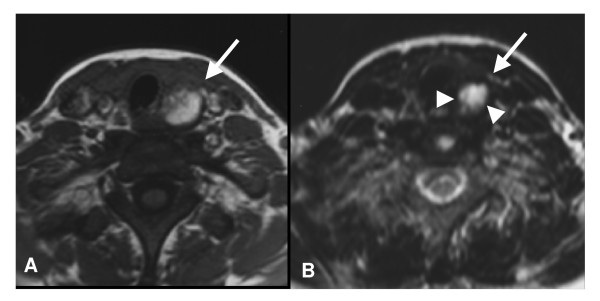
Left inferior parathyroid adenoma. Axial MR imaging scans (07/1998) weighted in T1 (A) and T2 (B) show a spontaneous hyperintense and well defined round lesion in both T1- and T2-weighted in the left-inferior parathyroid adenoma region (arrow). There is a hypointense lesion with a halo in the T2-weighted image which can represent degradation products of hemoglobin (arrowheads).
